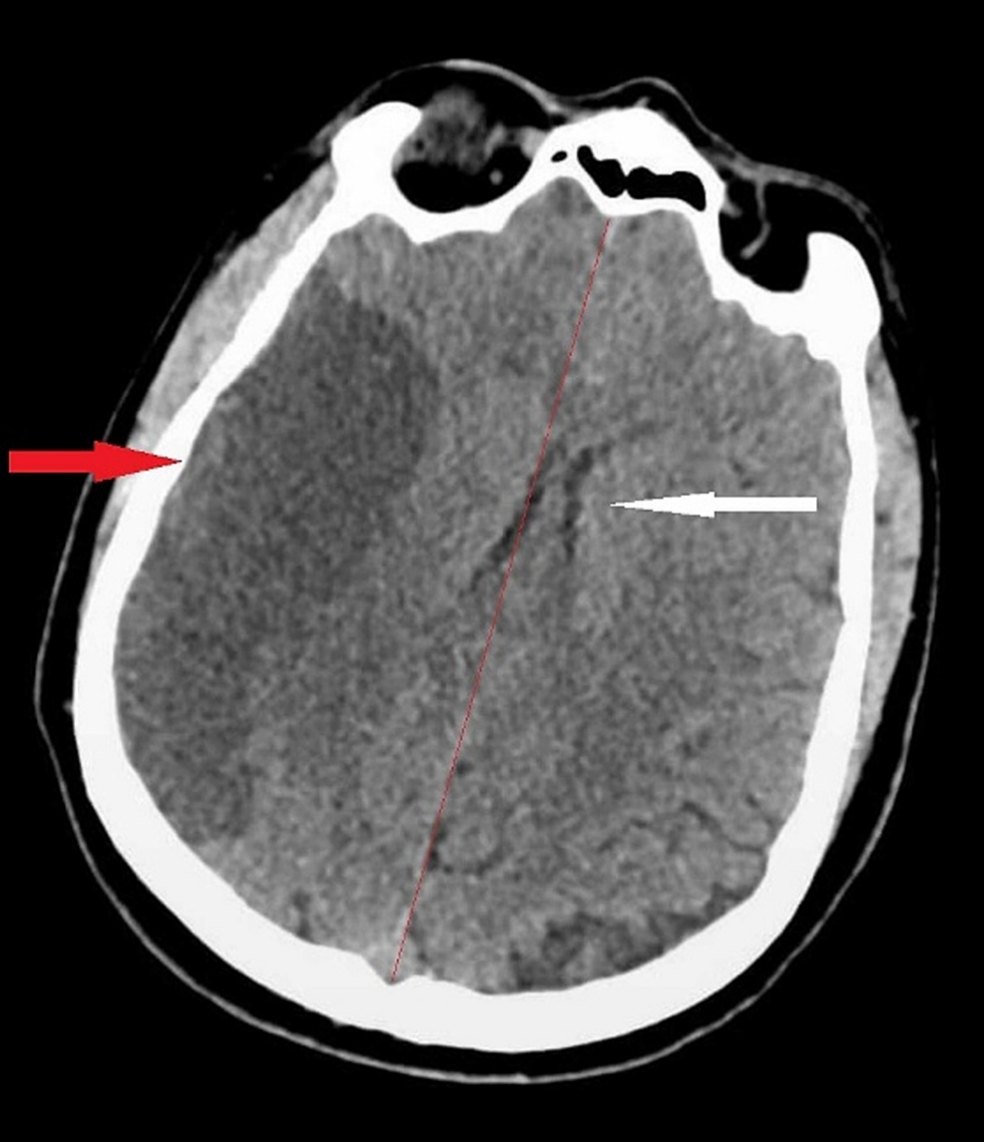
Non-contrast CT head on day 3. Red arrow depicts the ischemic area extending through fronto-parietal and temporal areas. White arrow shows mass effect in the form of effacement of the adjacent cortical sulci and ipsilateral lateral ventricle with midline shift towards the left side of 5.5 mm CT: Computed Tomography
